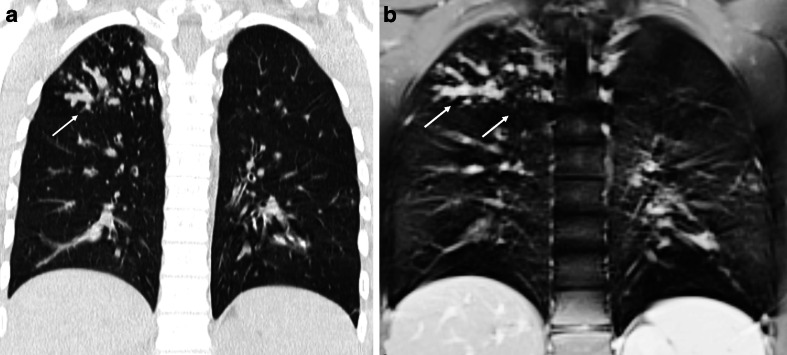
MRI with radial k space trajectory in cystic fibrosis. a CT versus (b) coronal free-breathing MRI with radial k space trajectory (PROPELLER) in a 21-year-old man with cystic fibrosis. There are areas of mucus plugging and bronchiectasis in the right upper lobe (*arrows*)
